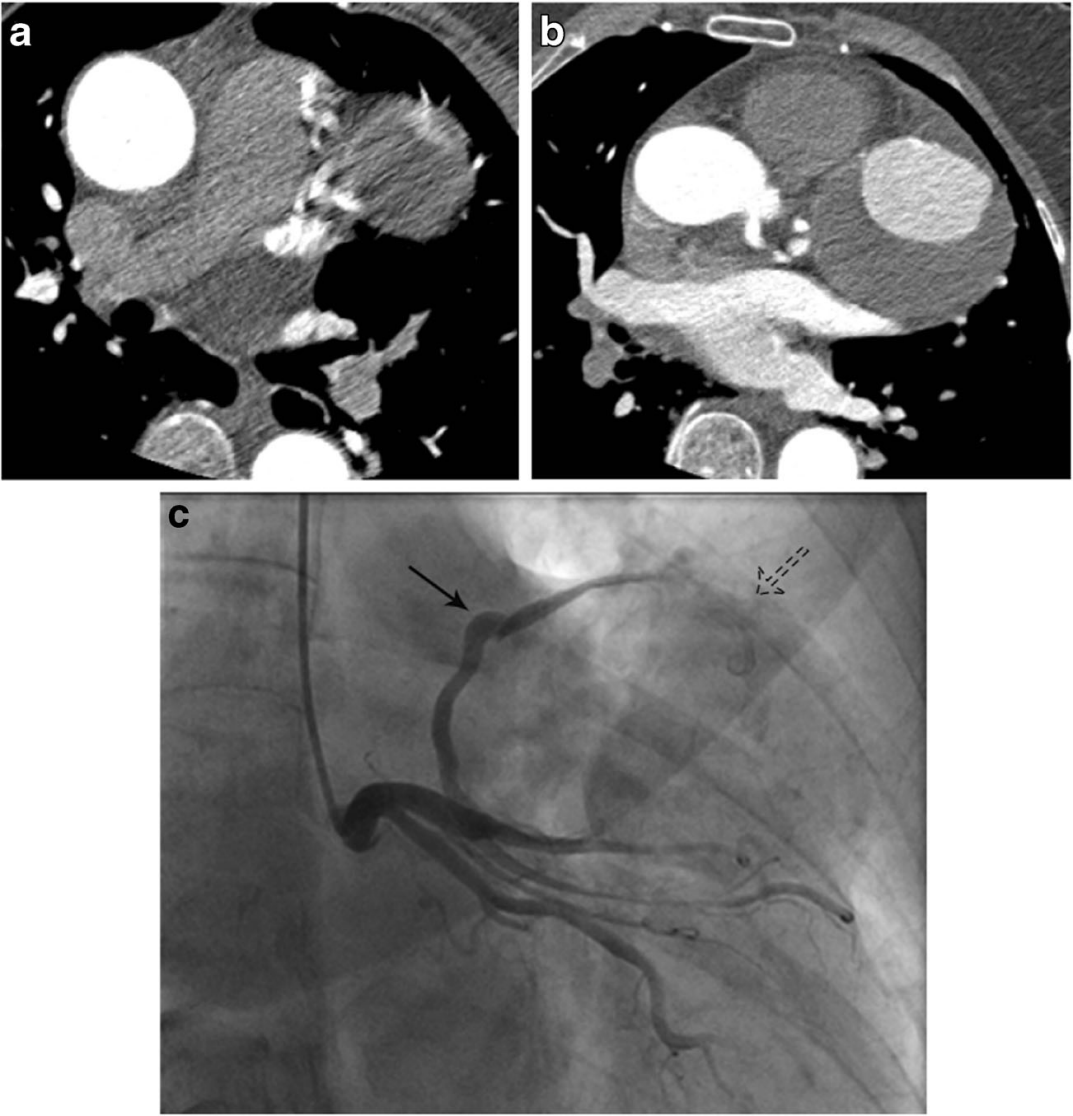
Axial CT images from a patient with coronary artery to pulmonary artery fistula, which results in partial opacification of the main pulmonary artery while contrast is maximally opacifying the aorta, seen on axial CTA (a, b) and conventional angiography (c). On conventional angiography, the fistula is seen opacifying on the arterial phase (*arrow*). The result is a systemic to pulmonary artery shunt. Notice the large thrombus in the A-V malformation abutting the main pulmonary artery
